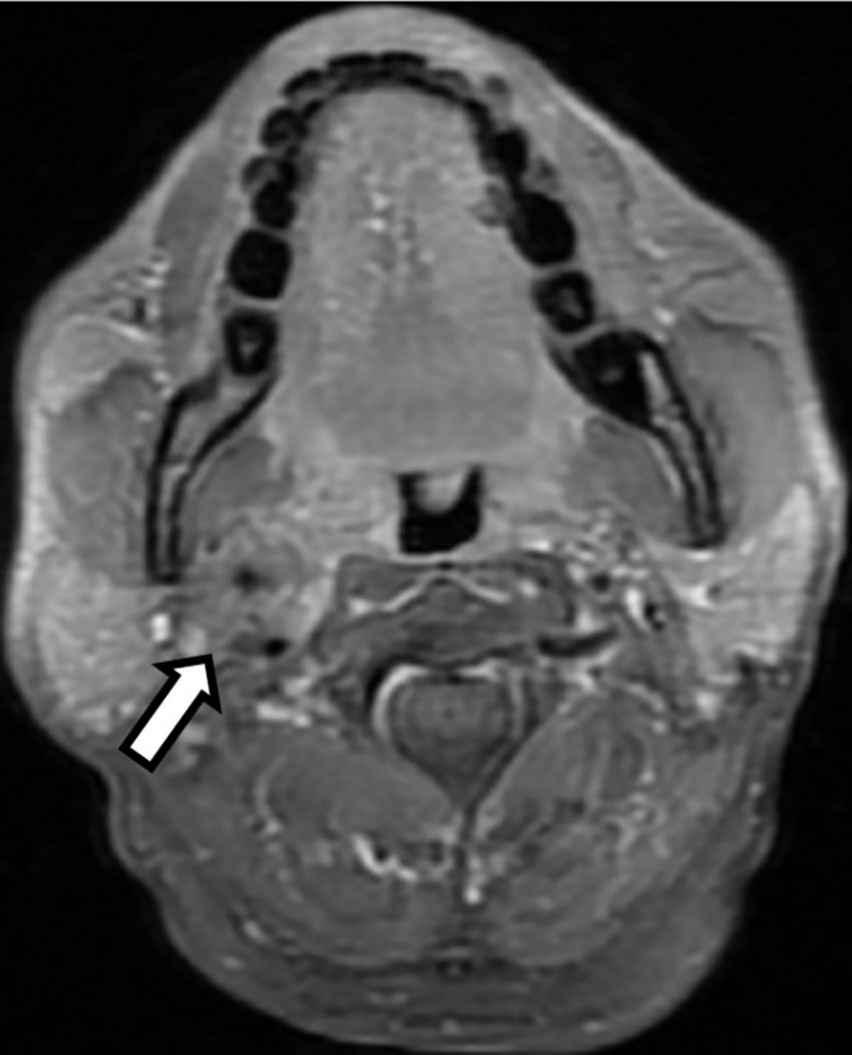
T1-weighted head and neck magnetic resonance image (MRI) with fat saturation revealed a 2.6 centimeter enhancing soft tissue lesion occupying the right carotid space and the right parapharyngeal space at the level of carotid bifurcation with right internal and external carotid artery encasement (Open arrow).
